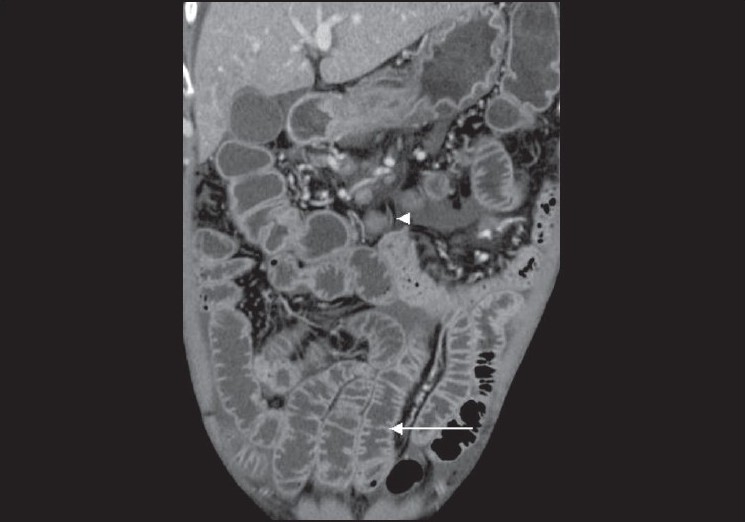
Coronal CT scan shows increased number of folds in the ileum (arrow) with mesenteric lymphadenopathy (arrow heads)
